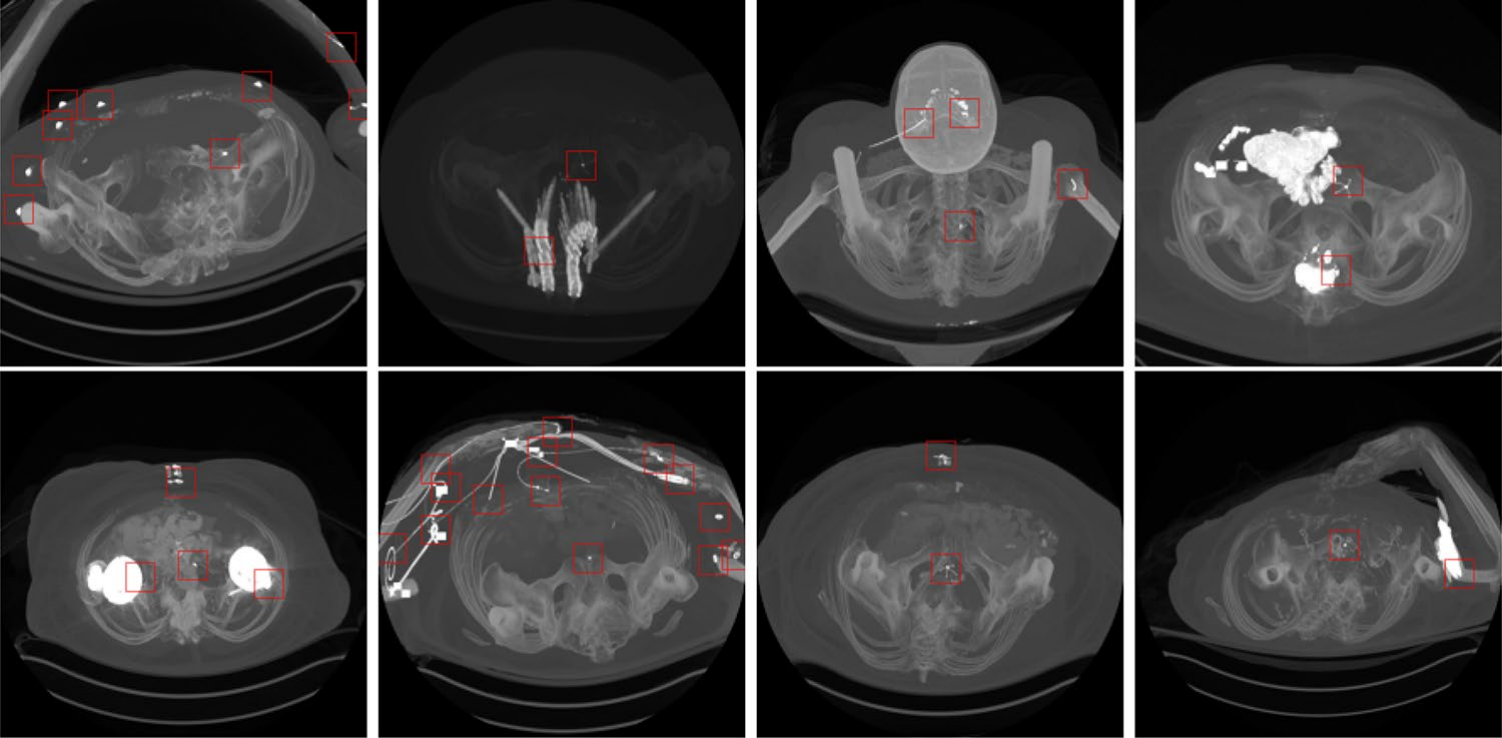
Example metallic objects in the abdominal CT (indicated in red bounding box), which have higher attenuation value than the predetermined threshold attenuation value for metallic objects. Several metallic objects can be present in the abdominal CT scan, such as surgical clips, intravascular stents, spinal fusion hardware, retained shrapnel, or swallowed foreign-bodies
